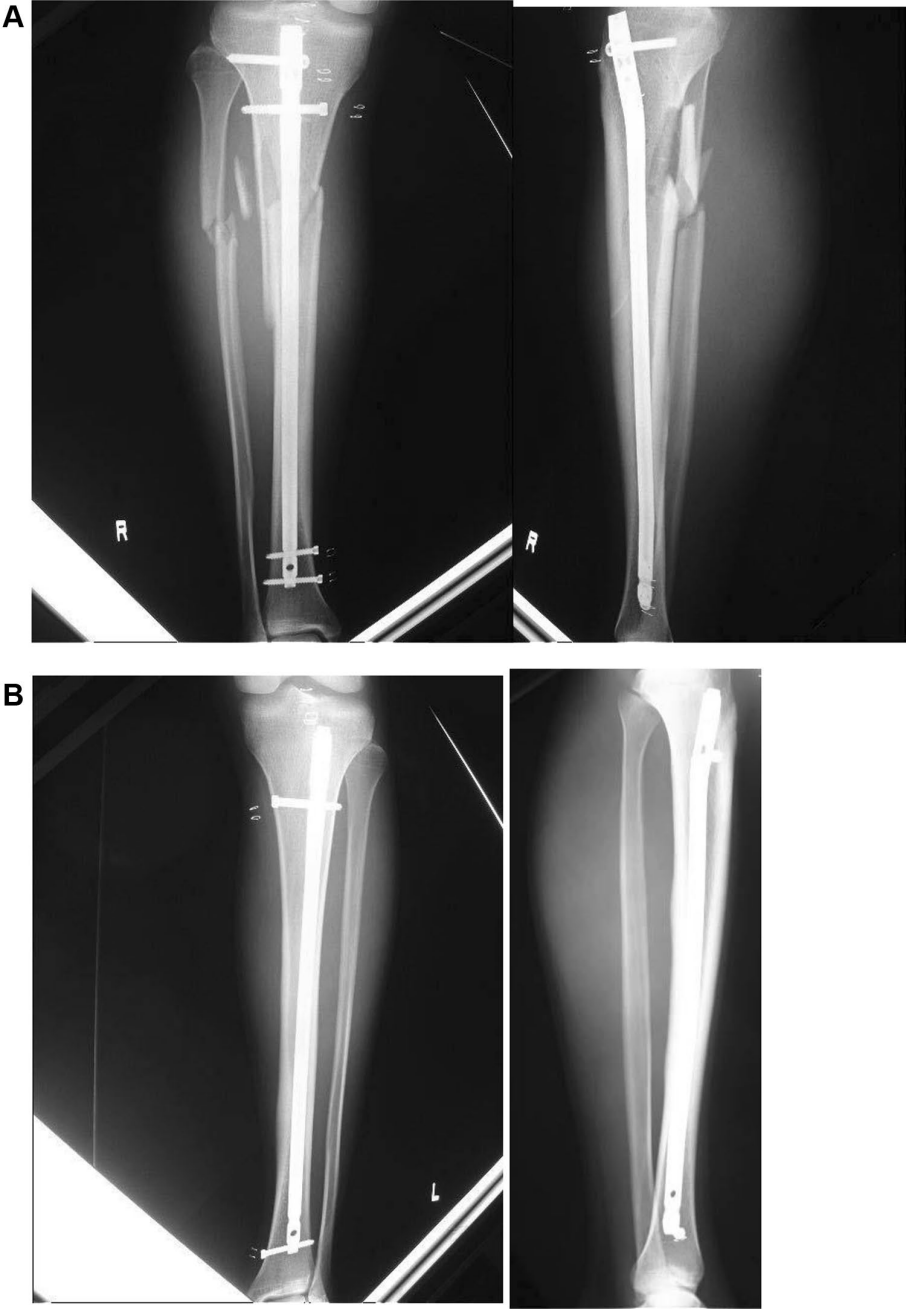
Radiographs show fixation of both tibial fractures, complete and incomplete, with intramedullary nailing. Closed reduction and internal fixation of the radial shaft fracture were performed using intramedullary nailing for both the right complete tibial fracture (A) and the left tibial stress fracture (B)
